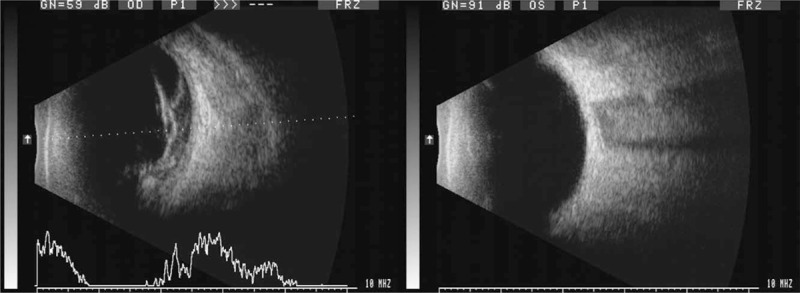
B-scan ultrasound images displayed the “table top” shaped tractional retinal detachment (TRD) in the right eye and partial pre-retinal fibrous membrane in the left.
